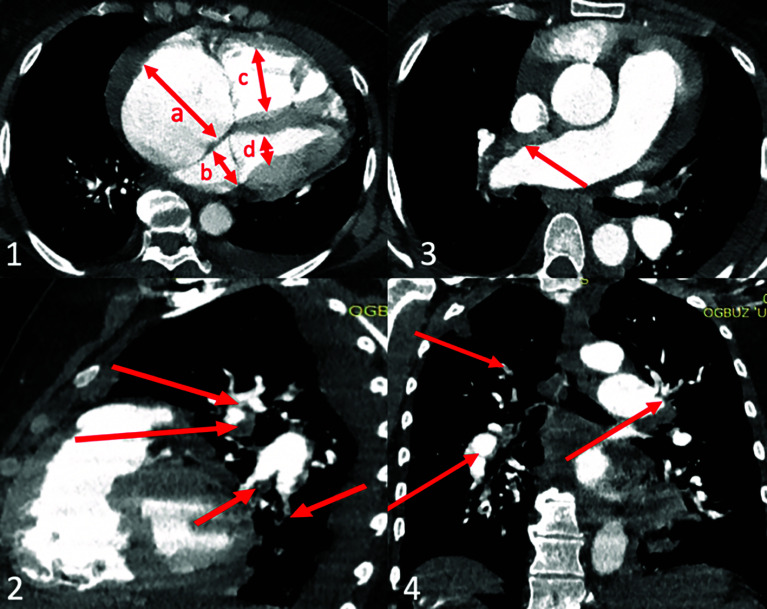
CT-angiogram: 1 – axial view shows right atrium (a) and right ventricle (c) enlargement compare to left atrium (b) and left ventricle (d); 2 – sagittal view shows organized thrombi in lobar and segmental branches of left pulmonary artery (red arrows); 3 – axial view shows proximal organized thrombus in right main pulmonary artery (red arrow); 4 – coronal view shows organized thrombi in right and left lobar pulmonary artery branches (red arrows).
